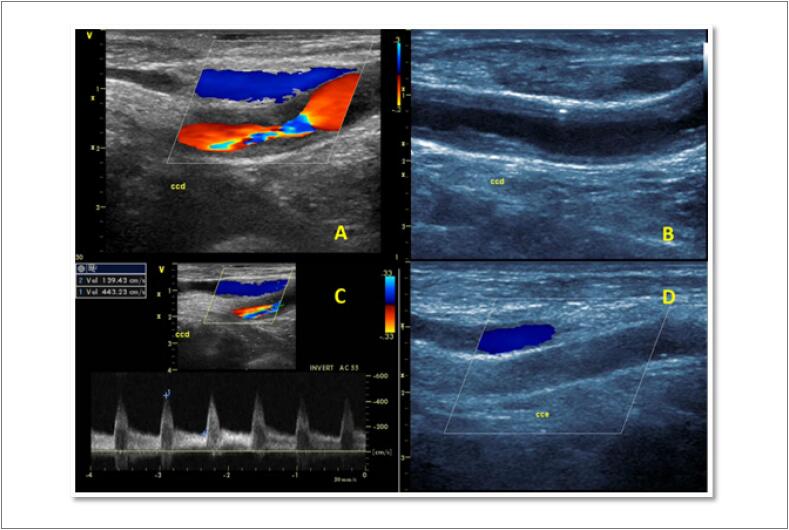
Paciente com arterite actínica, 6 anos após a radioterapia. A. Estenose maior que 70% da artéria carótida comum direita ao Doppler colorido – observa-se grande redução luminal e turbulência do fluxo. B. Artéria carótida comum direita com grande aumento da espessura mediointimal (EMI) ao modo B causando com redução luminal. C. Doppler pulsado com elevação da velocidade de fluxo. D. Carótida comum esquerda ocluída.
